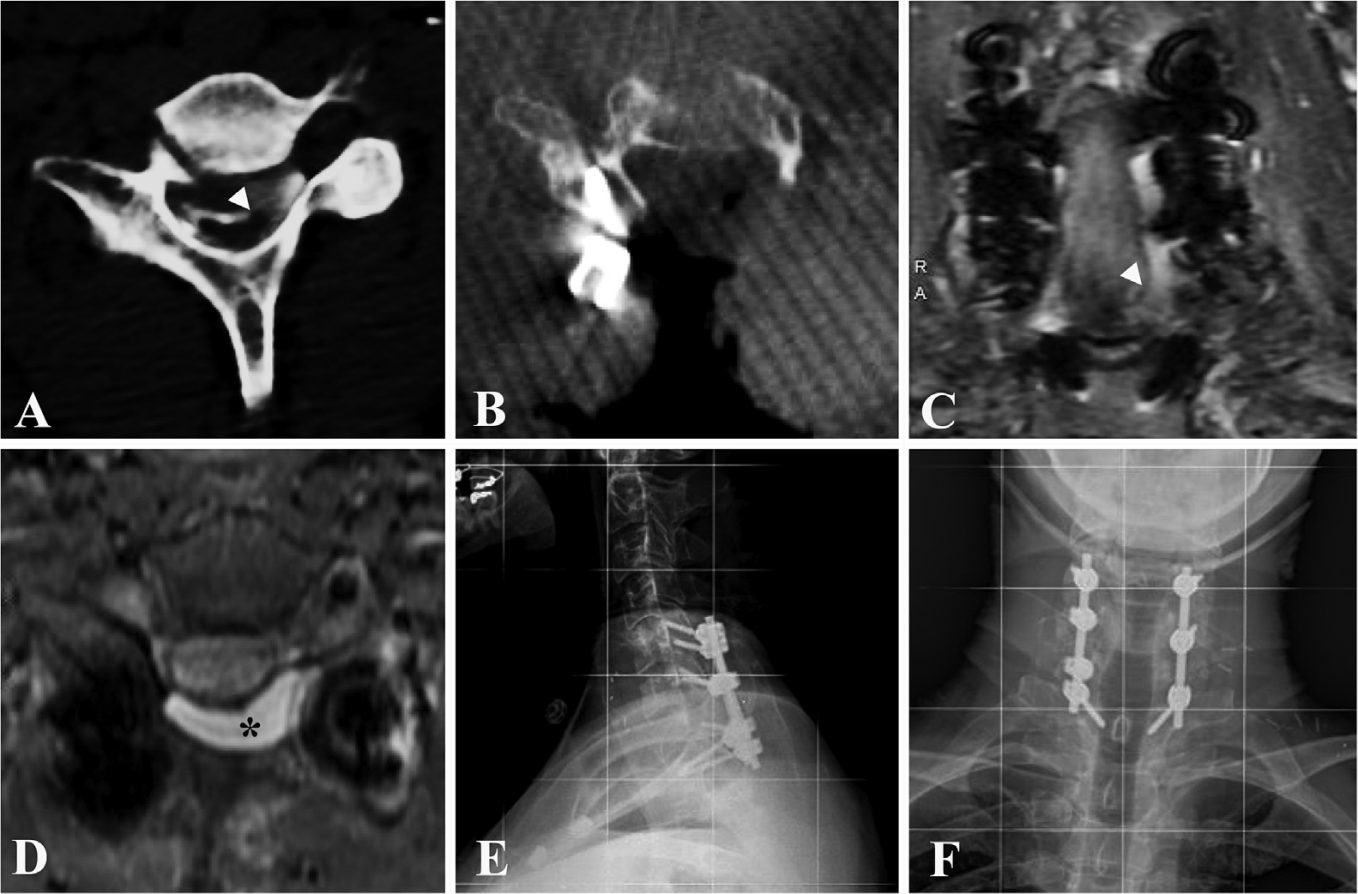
Panel A: Axial cut of a preoperative CT myelogram showing spinal cord herniation (SCH) through a dural defect after C8 nerve root avulsion (white arrowhead), and compression due pseudomeningocoele. Panel B: Axial cut of an intraoperative CT scan at the same level as panel A showing right-sided lateral mass screw, and extent of bony resection completed to allow dural repair (fascetectomy, pedicular resection). Panel C: Coronal view, T2-weighted sequence, of a postoperative MR neurogram. Instrumentation artifact and previously repaired BPAI-SCH with absence of nerve root sleeve pseudomeningocoele (white arrowhead) are visualized. Panel D: Axial cut of MR neurogram (T2 sequence) showing postoperative seroma (black asterisk), instrumentation artifact, and repaired BPAI-SCH with absence of nerve root sleeve pseudomeningocoele. Panels E and F: Postoperative lateral and anteroposterior plain XR, respectively, showing instrumentation in place.
